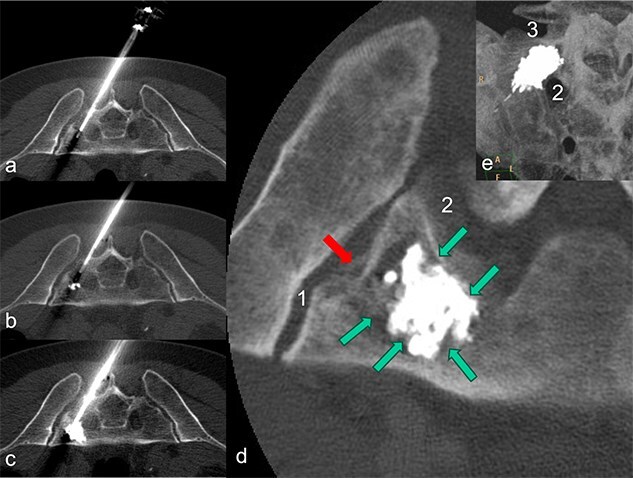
The patient is lying prone in the CT. The needle of the application system (a) was inserted from the dorsal side via the short axis. During the cement insertion, the successive, discontinuous filling of the metastasis-related defect zone in the right lateral mass of the sacrum is shown in (a) to (b). The highly viscous cement activated by radio frequency enables precise augmentation while simultaneously blocking a leak. In (d), the inserted PMMA cement is placed centrally in the defect zone as a cement seal (marked by green arrows); there is no cement leakage through the pathological fracture (marked by a red arrow) in the direction of the sacroiliac artery (1) and the neuroforamen (2). A MIP-reformation (d) shows the cement filling, whereby here too there is no leakage in the direction of the neuroforamen (2) and the adjacent disc space (3).
